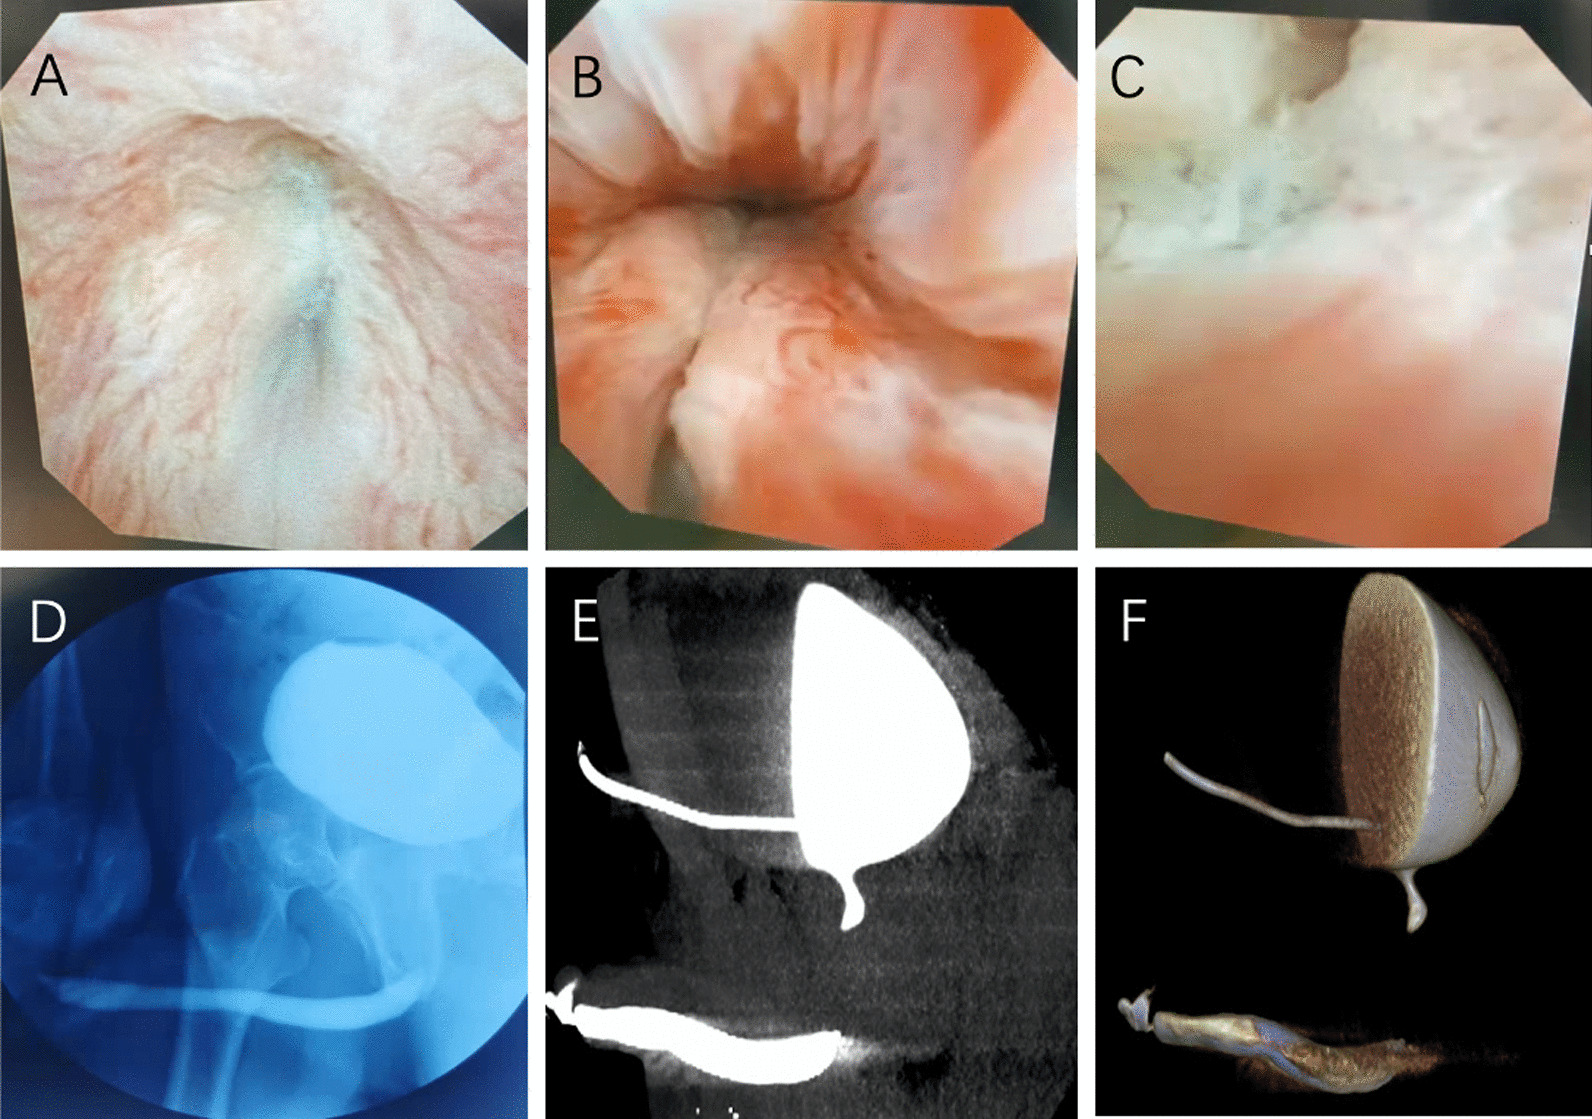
Various methods of urethral evaluation A distal end of urethra in patients with urethral stenosis under urethroscope; B Bladder neck of patients with urethral stenosis under urethroscope; C Proximal end of urethra in patients with urethral stenosis under urethroscope; D Urethrography showed urethra and bladder; E CT scan of urethra and bladder in sagittal plane; F 3D reconstruction of the urethra and bladder from CT scans
